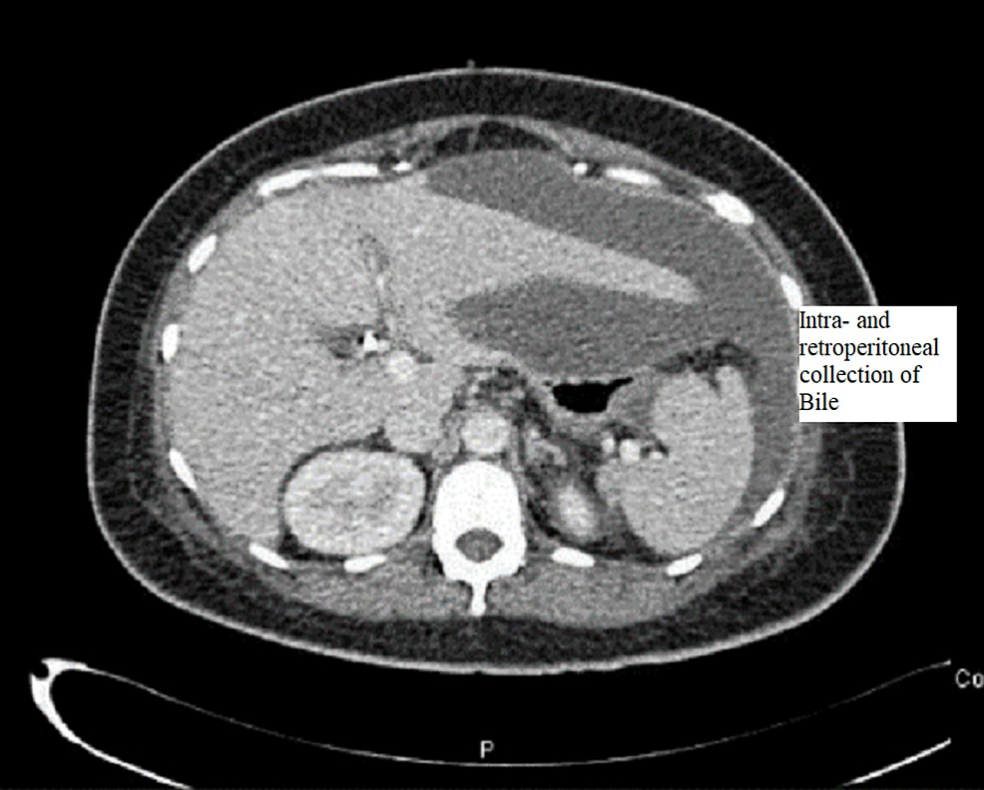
CT abdomen and pelvis with contrast showing the free fluid collection in peri-hepatic and peri-splenic areas
